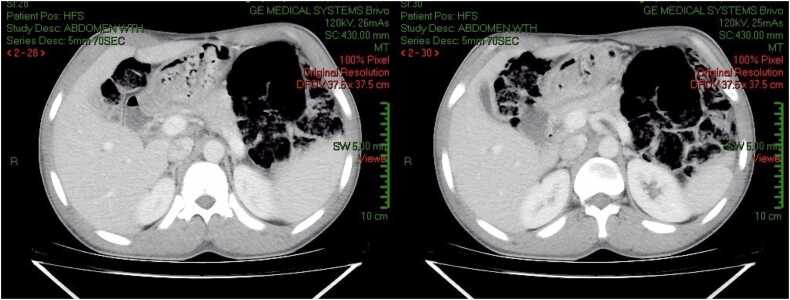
CT Scan: Marked intra and extra-hepatic biliary duct dilation improvements with slight residual dilation. No visible ductal lesions (Slice 28,30).
